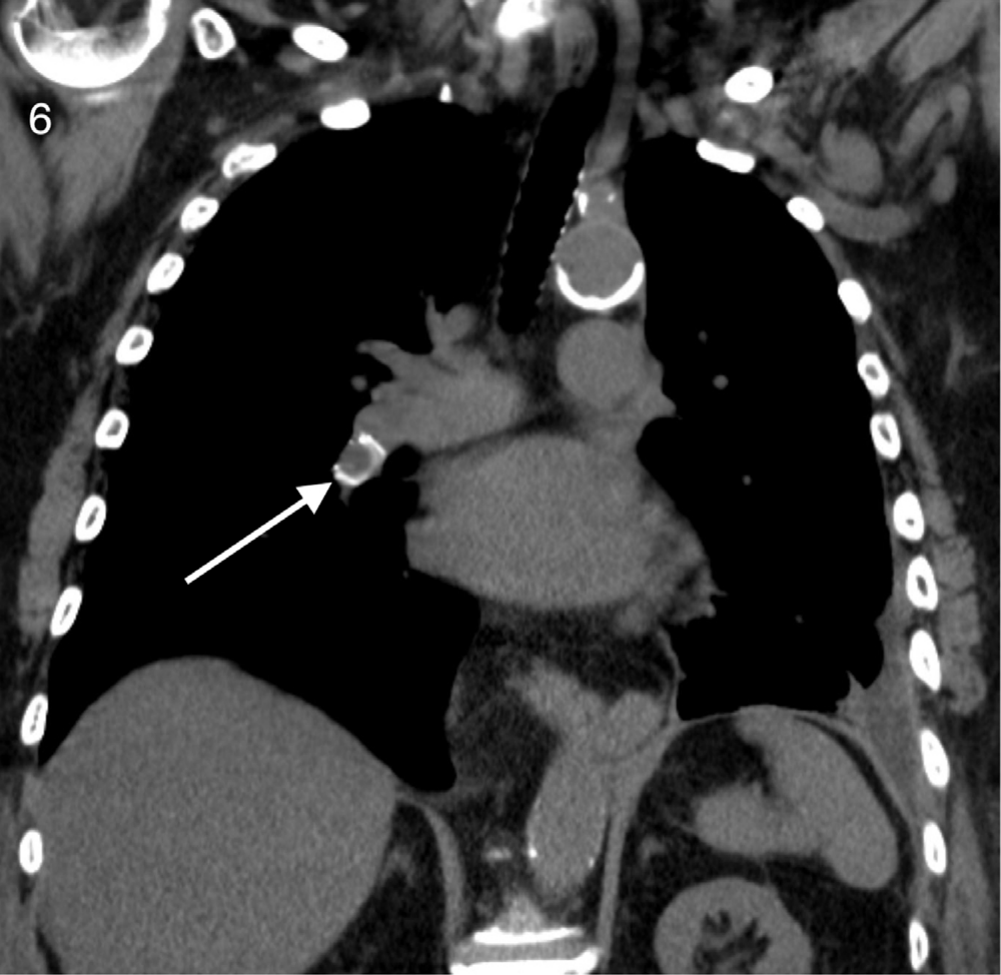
Coronal view CT demonstrating the second migrated stent in the right interlobar pulmonary artery (white arrow).
